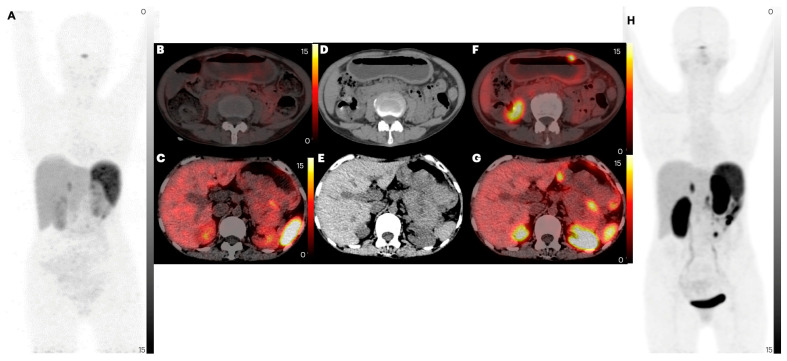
PET/CT images of patient number 17, a 44-year-old female with a known case of NET stomach grade 3, for whom on-table swallowing of water was undertaken for dilatation of stomach for clear visualization of the lesions. Images (A,H) are MIP images of [^68^Ga]Ga-DOTANOC and [^68^Ga]Ga-DATA^5m^-LM4 PET/CT, respectively. Images (B,C) are [^68^Ga]Ga-DOTANOC PET/CT axial images. Images (D,E) are CT axial images. Images (F,G) are [^68^Ga]Ga-DATA^5m^-LM4 PET/CT axial images. Increased number of gastric (primary) foci of tracer uptake are noted in [^68^Ga]Ga-DATA^5m^-LM4 PET/CT images.
